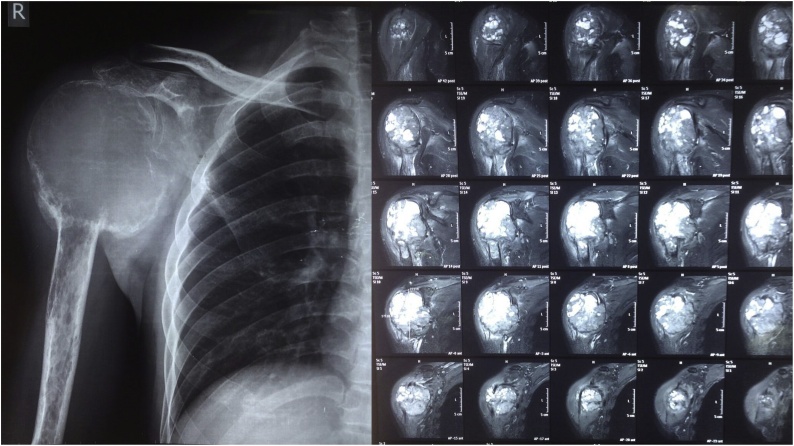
a).Plain radiograph, b).MRI.
